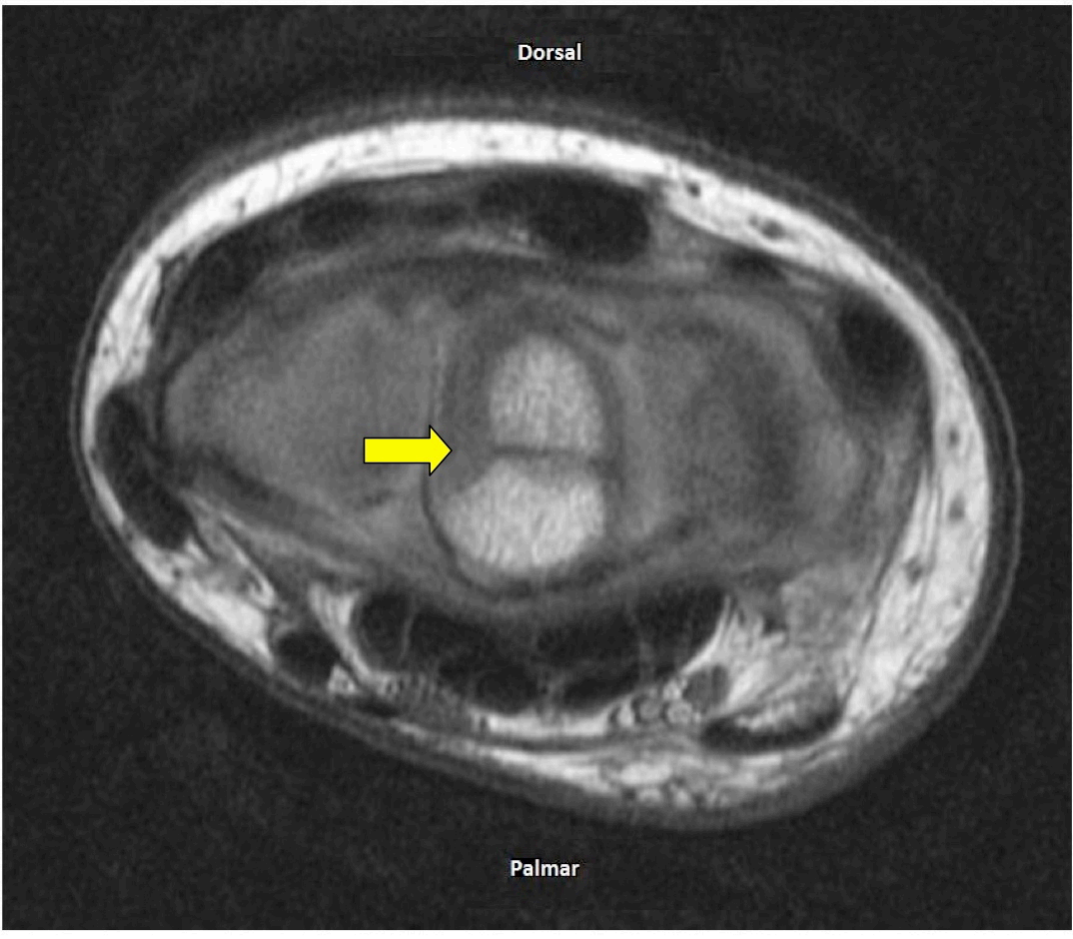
Axial T1-weighted magnetic resonance imaging (MRI) scan of the left wrist demonstrating the linear hypointense signal (arrow) between the dorsal and palmar parts of the lunate.
